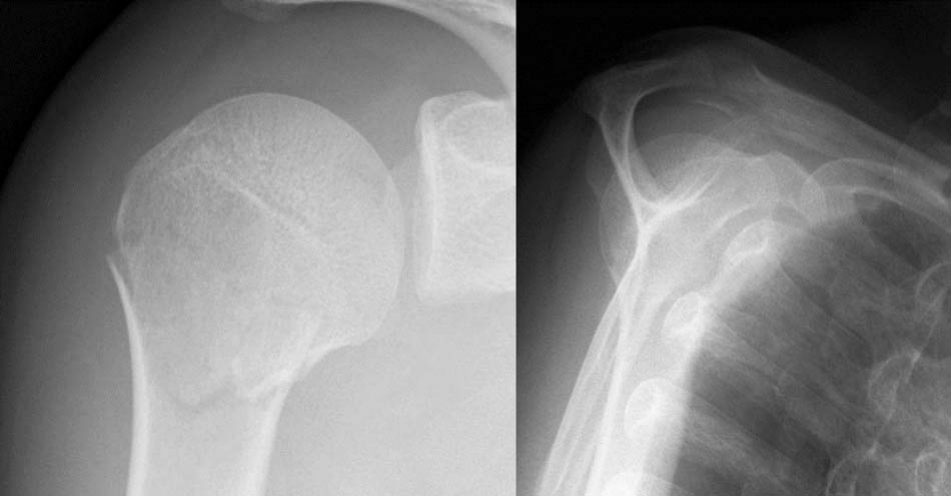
Original X-ray showing surgical neck fracture.
